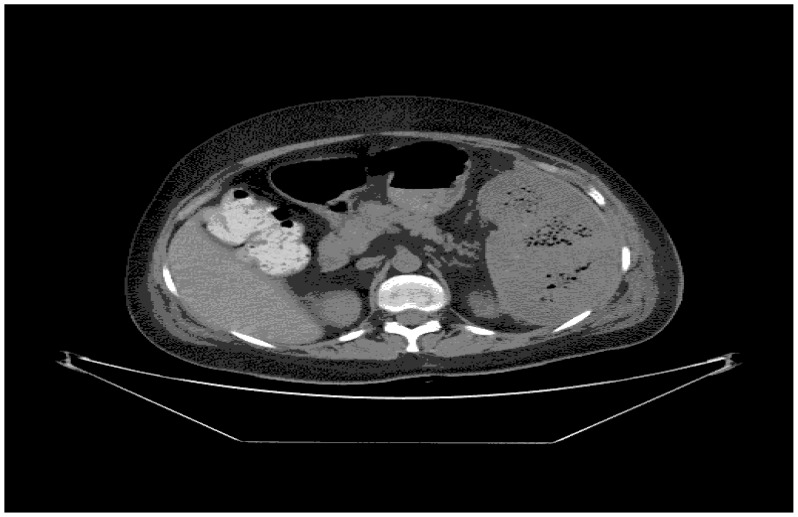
CT scan showing multiple infarcts in the parenchyma of the spleen.
